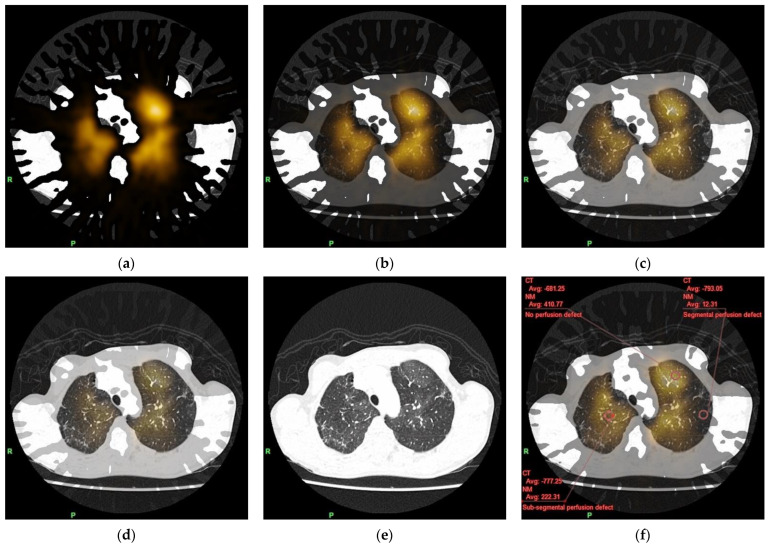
Fusion of lung subtraction CT and perfusion lung SPECT images at various blending ratios with and without ROIs placed in the three different classes of lung perfusion. Both a lung subtraction CT axial image at lung window display and a perfusion lung SPECT axial image can be fused by pixel-shift manual registration to eliminate spatial misregistration using the lung margin as the anatomical reference at various blending ratios, which can be arbitrarily determined from 0% ((a): perfusion lung SPECT only) to 100% ((e): lung subtraction CT only), including 25% (b), 50% (c), and 75% (d). Three circular ROIs of 1 cm^2^ can be manually placed in areas representing the three different classes of lung perfusion (Class 1, no perfusion defect; Class 2, subsegmental perfusion defect; and Class 3, segmental perfusion defect) on the axial fusion image at various blending ratios, such as 50% (f), for simultaneous (within each ROI) measurement of the CT value on lung subtraction CT and radioactive count on perfusion lung SPECT. ROI, region of interest; SPECT, single photon emission CT.
